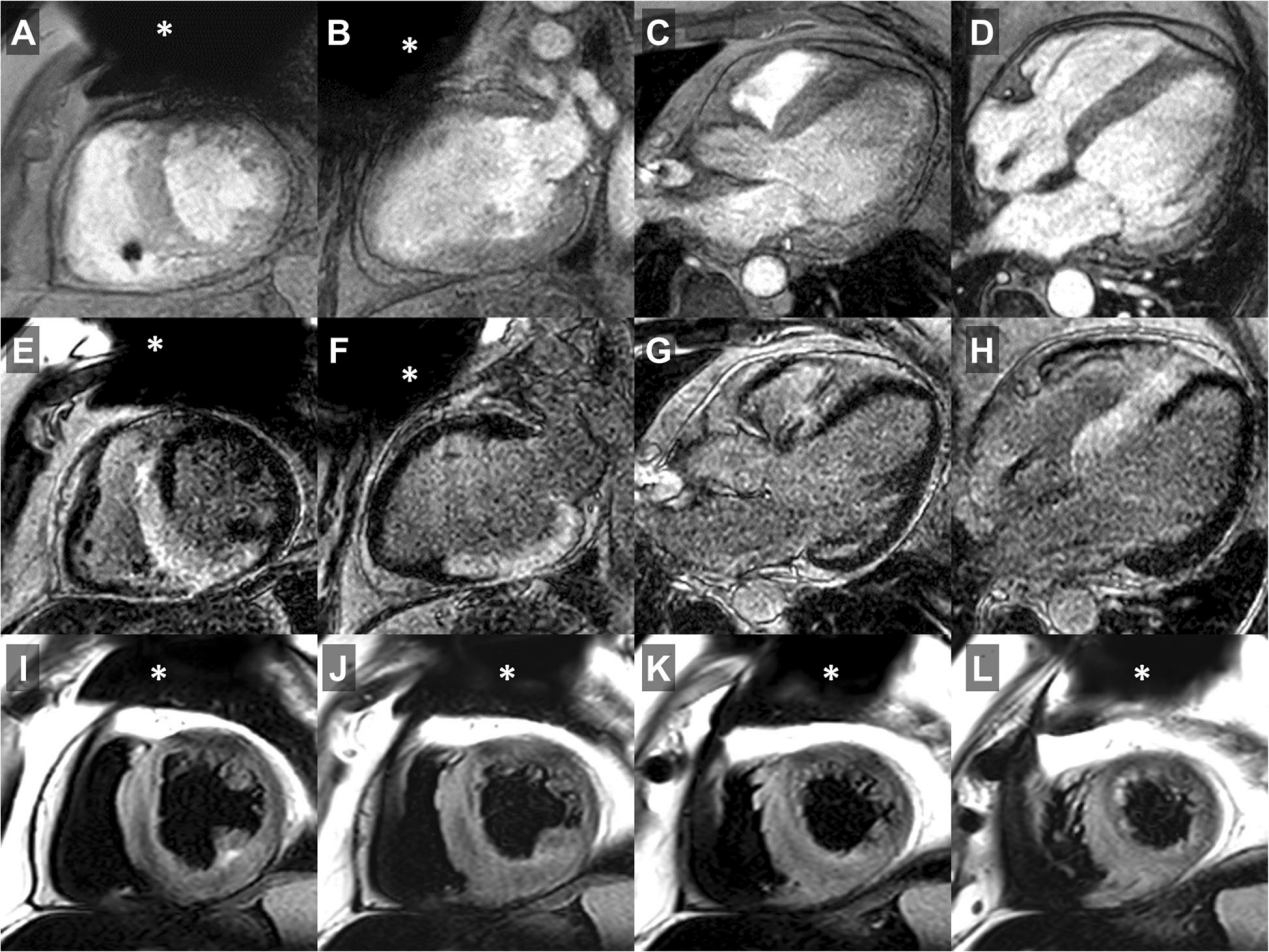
CMR imaging in a dual-chamber, secondary prevention ICD patient (male, 59 years) presenting with electrical storm (13 adequate shock episodes) 7.2 months after device implantation. Diagnostic change (+); management/treatment change (+): pre-CMR referral diagnosis: hypertensive heart disease; post-CMR diagnosis: acute disease state of cardiac sarcoidosis; pre-CMR treatment plan: ventricular tachycardia ablation; post-CMR treatment plan: medical therapy including steroids. A – D, post–contrast cine imaging in all cardiac standard geometries showed concentric left ventricular (LV) hypertrophy with mildly reduced global LV ejection fraction (LVEF 47%); E – H, LGE CMR imaging (identical geometries) identified extensive, mostly transmural areas of positive LGE in all septal and inferior LV segments with corresponding edema on T2-weighted turbo spin echo imaging (I – L, consecutive short-axis geometries) consistent with an acute inflammatory disease state of cardiac sarcoidosis subsequently being confirmed by endomyocardial biopsy. Approximate generator position is indicated by the asterisk
